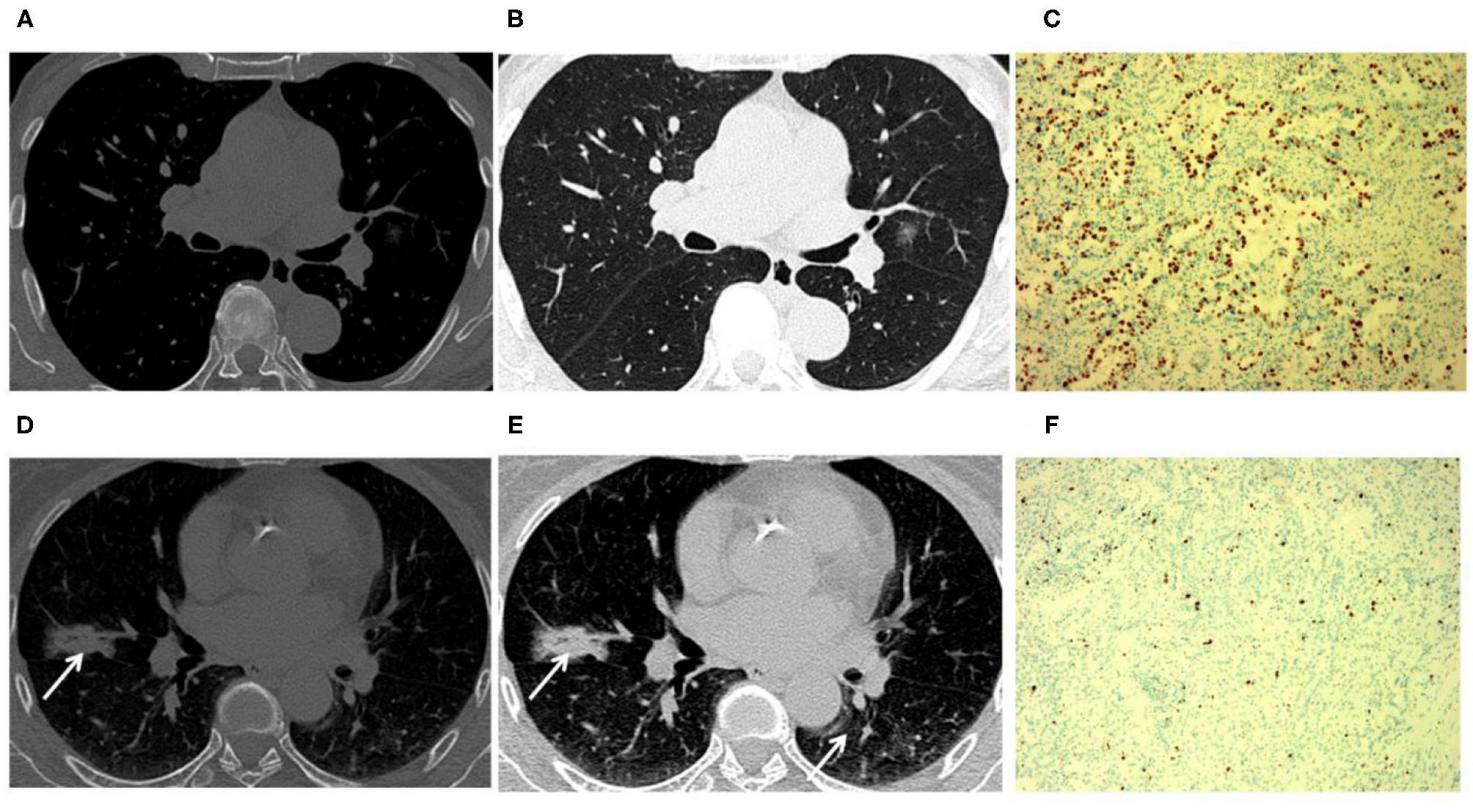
Tumors with positive Ki-67 expression levels were more inclined to have air bronchograms than those with negative Ki-67 expression levels. An 81-year-old male patient with Ki-67 negative expression (A–C). (A,B) CT image showed that a tumor had a negative expression of Ki-67 without an air bronchogram. (C) The Ki-67 expression was 5% in the glandular epithelium. A 63-year-old female patient with positive Ki-67 expression (D–F). (D,E) CT image showed that a tumor had positive Ki-67 expression with an air bronchogram. (F) The Ki-67 expression was 55% in the glandular epithelium (arrow).
